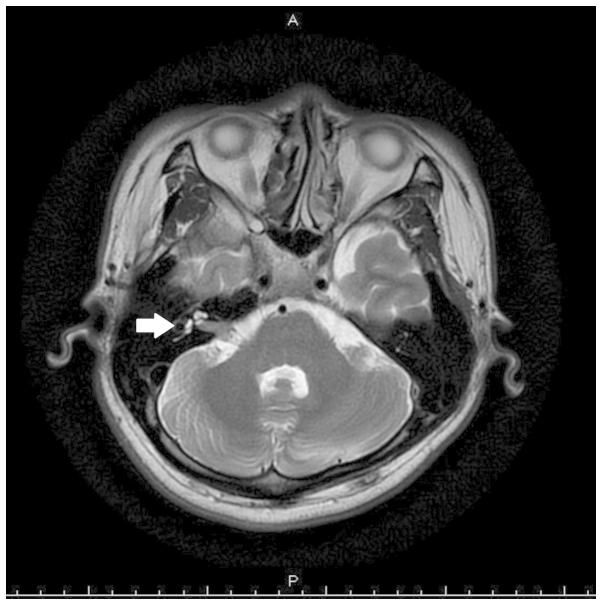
Contrast-enhanced T2-weighted magnetic resonance imaging reveals an intracanalicular tumor in the region of the cerebellopontine angle (shown by the arrow).
